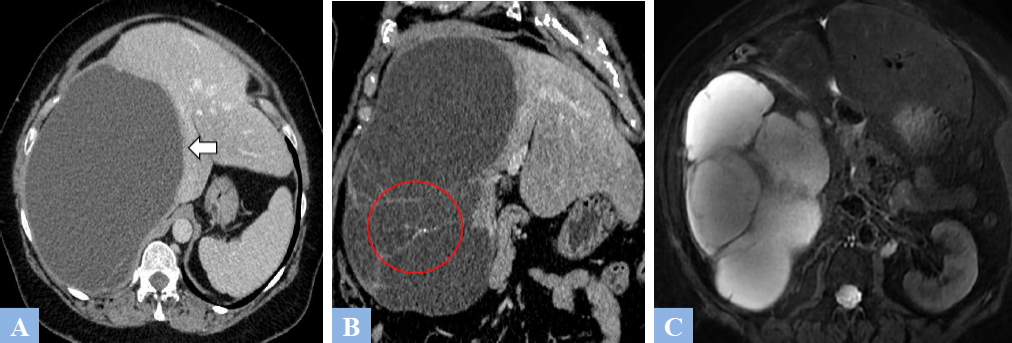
Mucinous cystic neoplasm in a 57-year-old female patient. CT and MRI features. A. CT image in the axial plane at portal phase of enhancement. Large cystic lesion in the right liver (arrow) displacing the remaining liver to the left. B. Same study, coronal reconstructions showing enhanced intracystic septations after contrast injection at the lower part of the lesion, associated with fine septal calcifications (red circle). C. T2-weighted fat-suppressed MR image in the axial plane. Septations appearing as T2 hypointense (red circle) within a T2 hyperintense fluid-filled cystic lesion.
